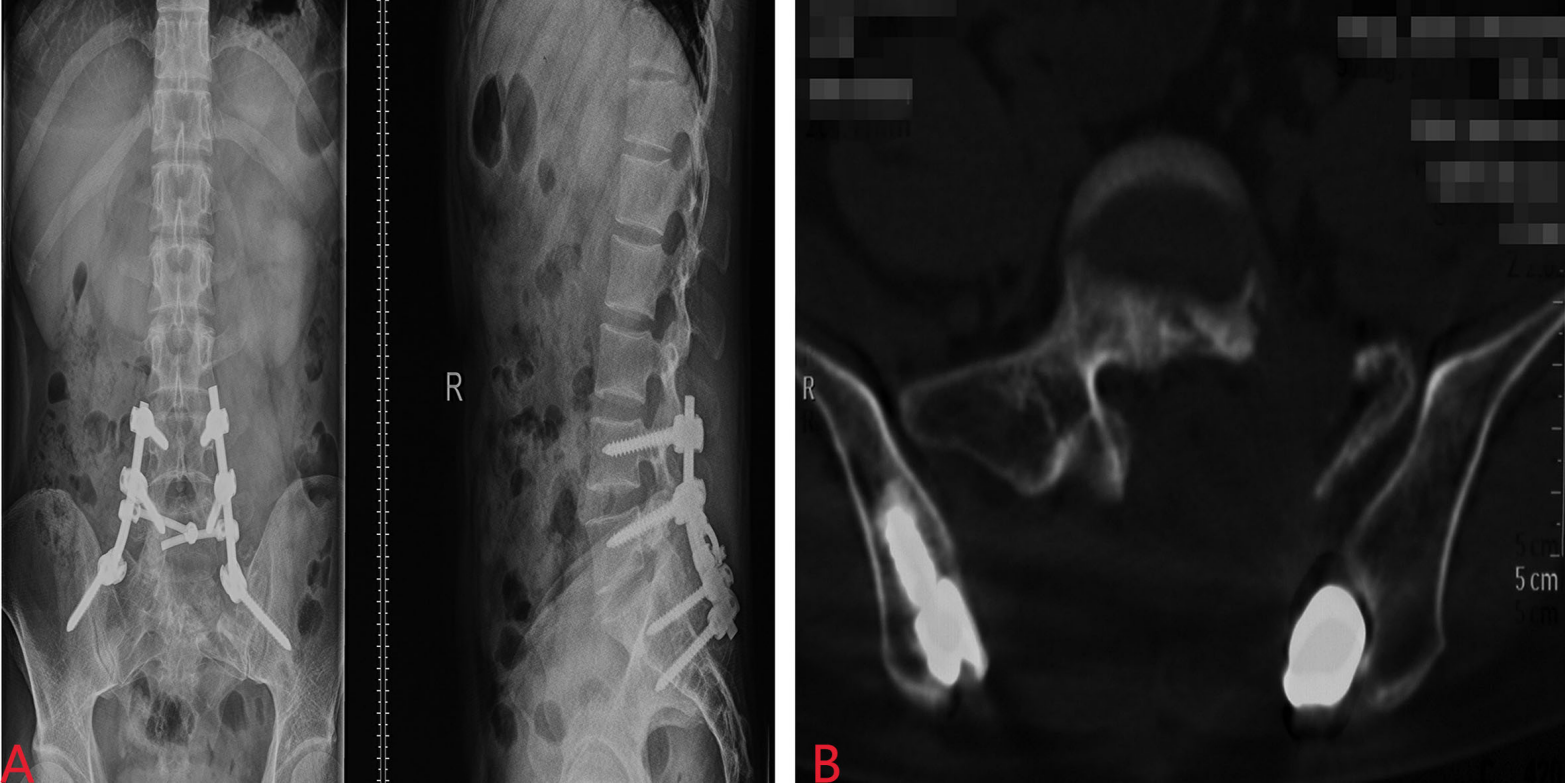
Postoperative X-ray (A) and CT (B) images did not indicate any change of the operative region.
